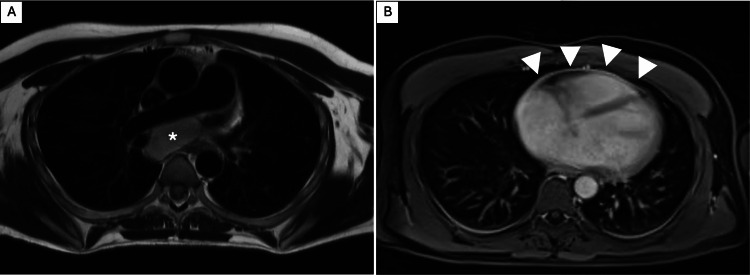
Magnetic resonance imaging (MRI) three weeks after treatment with amoxicillin-clavulanate and oral acetylsalicylic acid Axial T2-weighted image at the level of the subcarinal cyst (A) demonstrates a relatively high internal signal intensity consistent with fluid content (white asterisk), and a thin hypointense rim that enhanced after gadolinium (not shown). No internal septations or solid components are seen. Post-contrast T1-weighted VIBE (volumetric interpolated breath-hold examination) sequence with gadolinium at the level of the heart (B) reveals persistent mild pericardial thickening with associated linear enhancement (arrows), indicative of pericardial inflammation. The small pericardiac effusion has since resolved.
